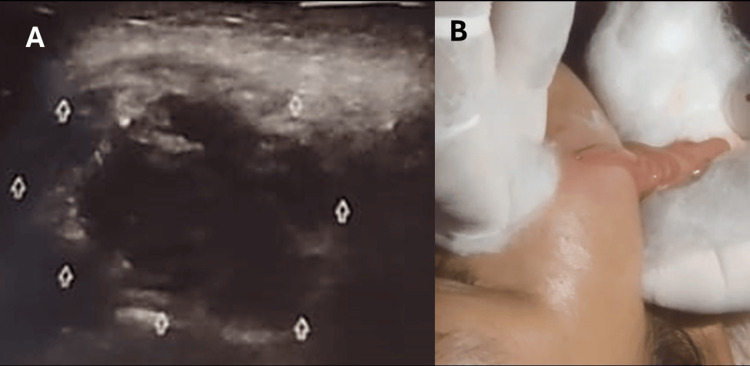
Ultrasound and surgical drainage of the facial abscess (A) Point-of-care ultrasound revealing a heterogeneous fluid collection (21.8 cc) in the right cheek. (B) Intraoperative image during incision and drainage of the abscess cavity.
